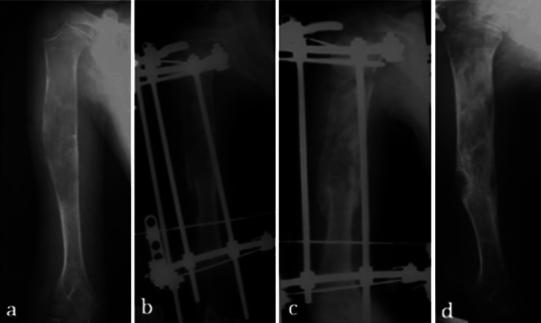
a X-ray of unicameral bone cyst (UBC) of right humerus in an 11-year-old boy. b Application of external fixator and first-level osteotomy with coaptation. c Three months after filling the distal part of the cystic cavity, we performed a second proximal-level osteotomy and distraction. d Capanna grade 2 healing with residual cavity
